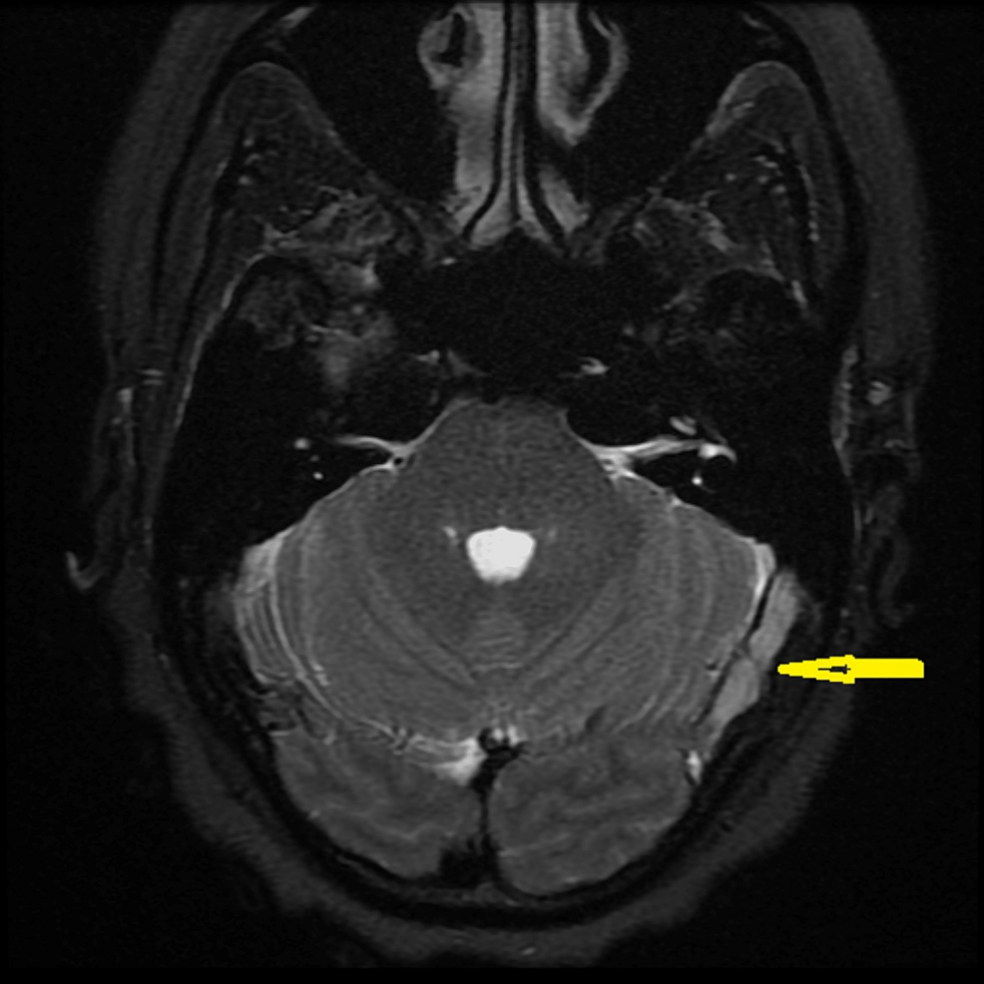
MRI head showing left transverse sinus thrombosis (yellow arrow)
